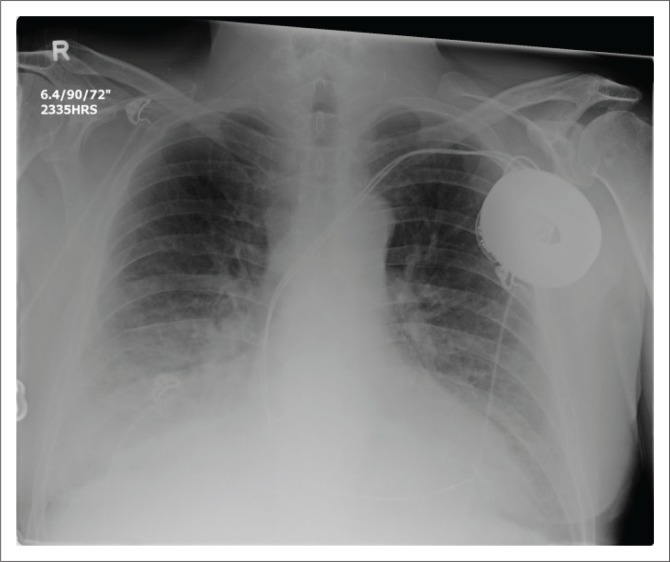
A chest radiograph showing a doughnut magnet placed over a pacemaker.
